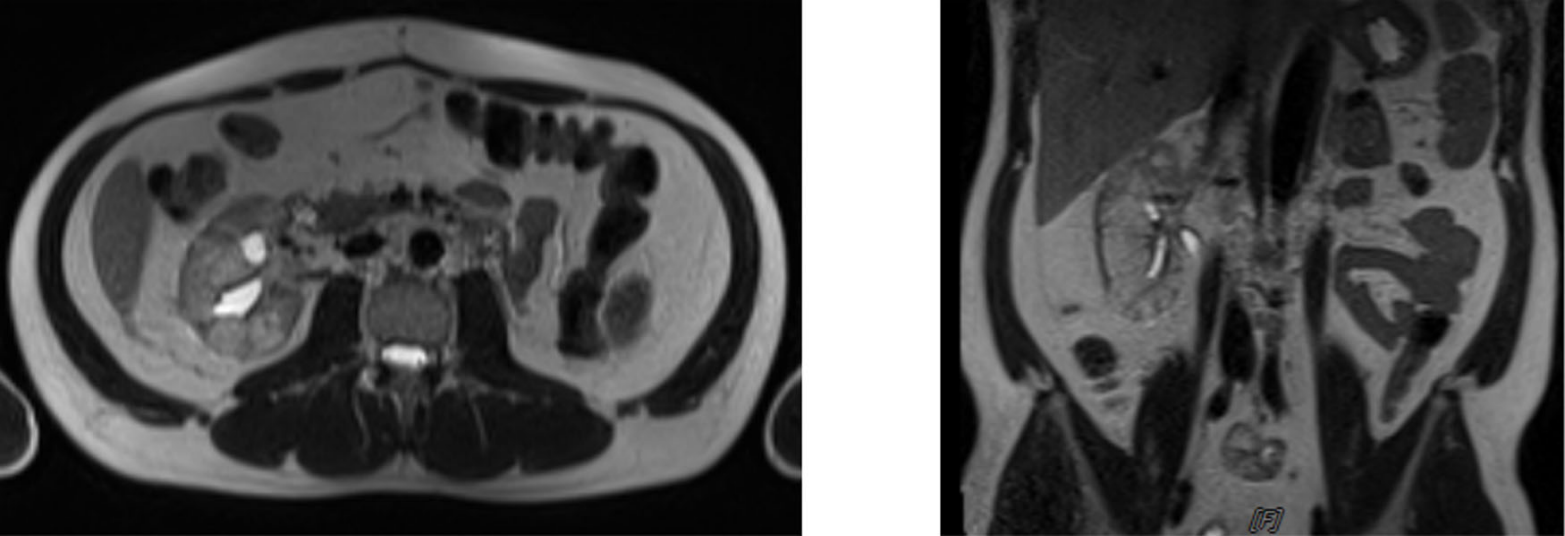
Abdominal magnetic resonance imaging (MRI) May 2021 shows benign calyx dilatation suspicious due to stricture, heterogeneous renal parenchyma.
